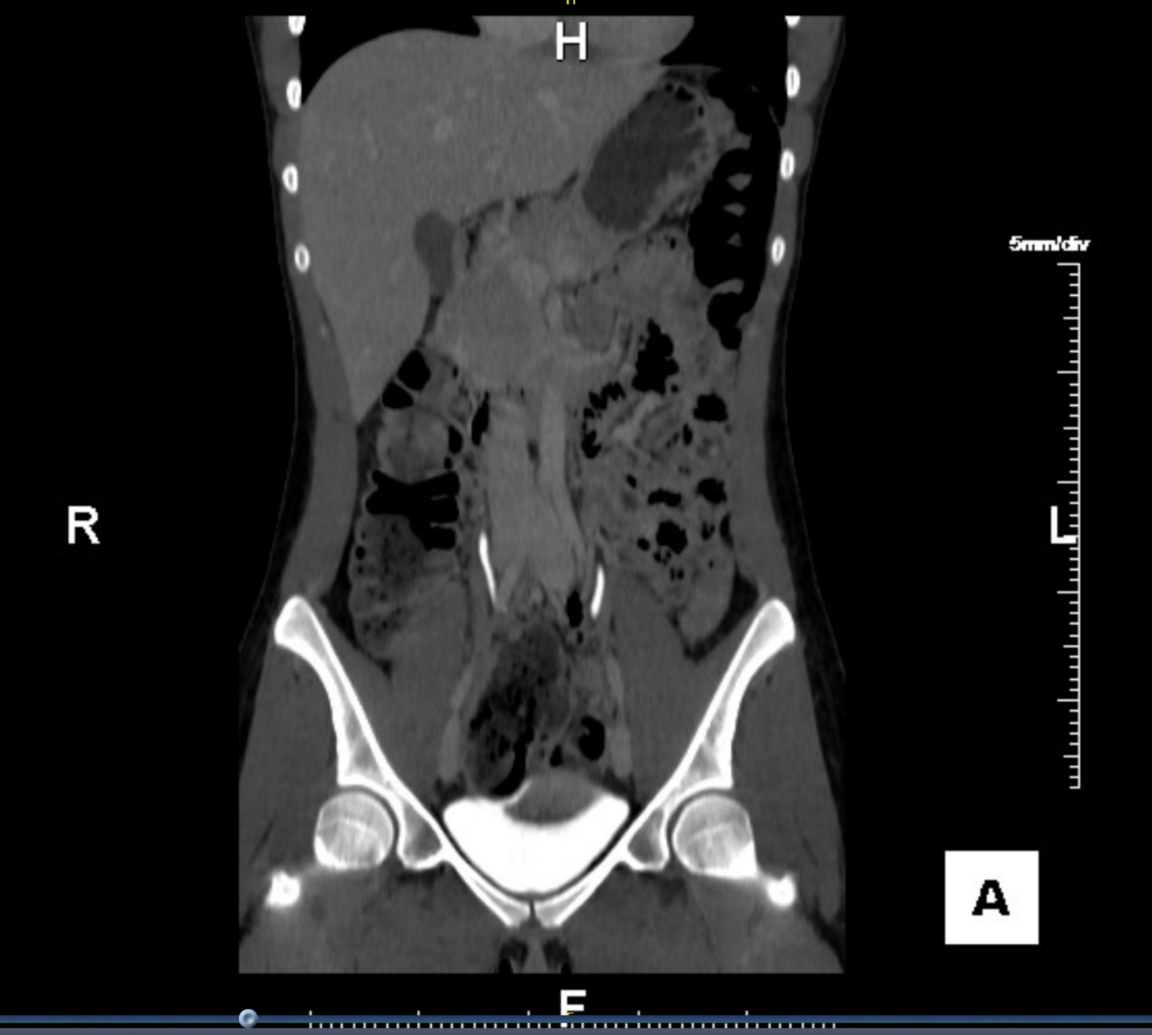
Normal abdominal and pelvic CT scan - coronal view CT: computed tomography
